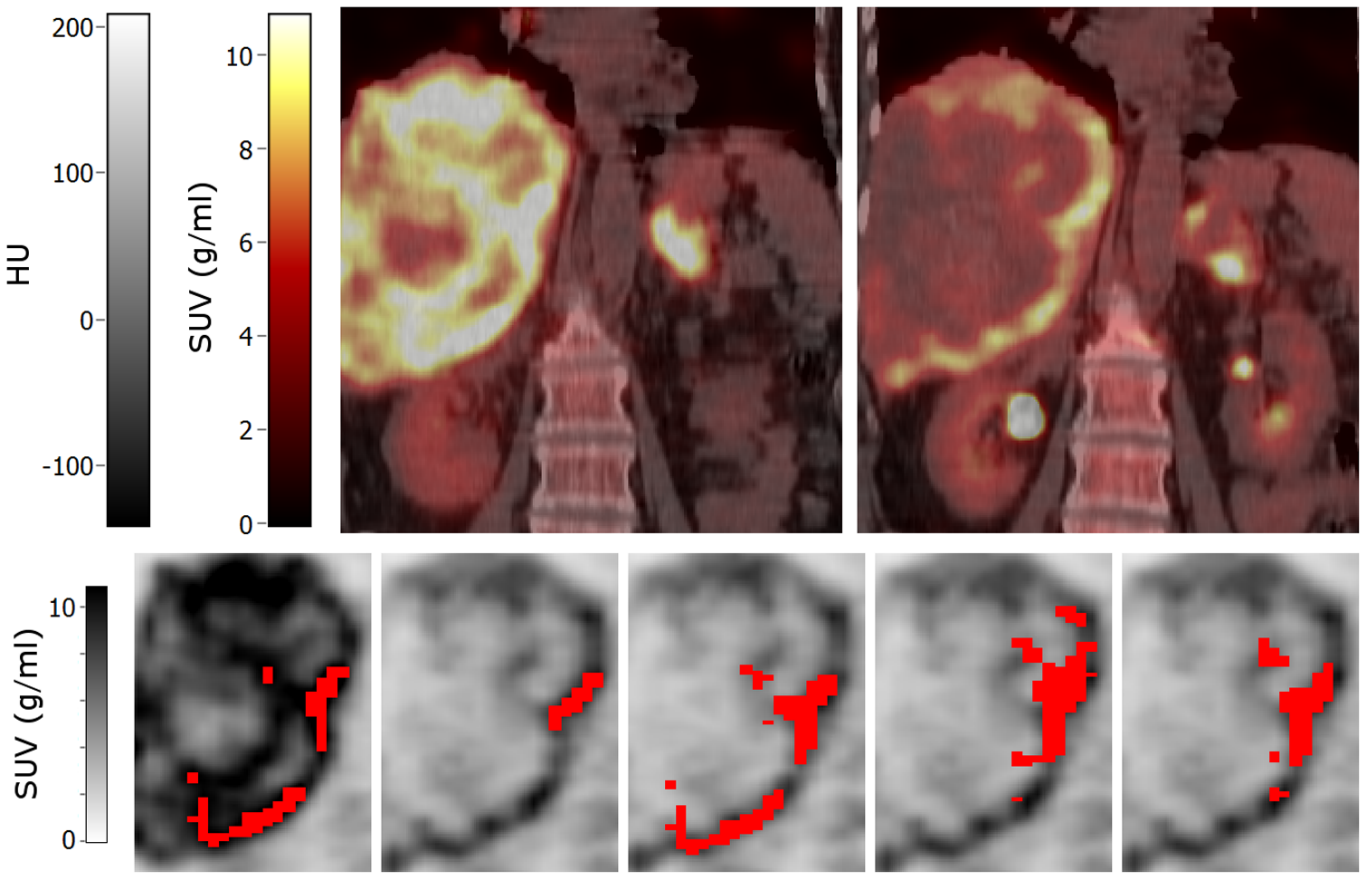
Coronal images of a patient with a large liver metastasis showing heterogeneous tracer uptake. Top row: baseline (left) and early response (right) PET/CT images. Bottom row: volumes of interest (shown in red) projected onto the baseline (first image) and early response scans (other images) that were obtained using (from left to right): A50 defined on baseline scan, A50 defined on early response scan, and local rigid PET, non-rigid PET and non-rigid CTPET image registration. All images are shown using the same colour scales. Abbreviations: SUV, standardized uptake values; HU, hounsfield units; A50, 3 dimensional (semi-)automatic isocontour method at 50% of the maximum pixel value that corrects for local background.
